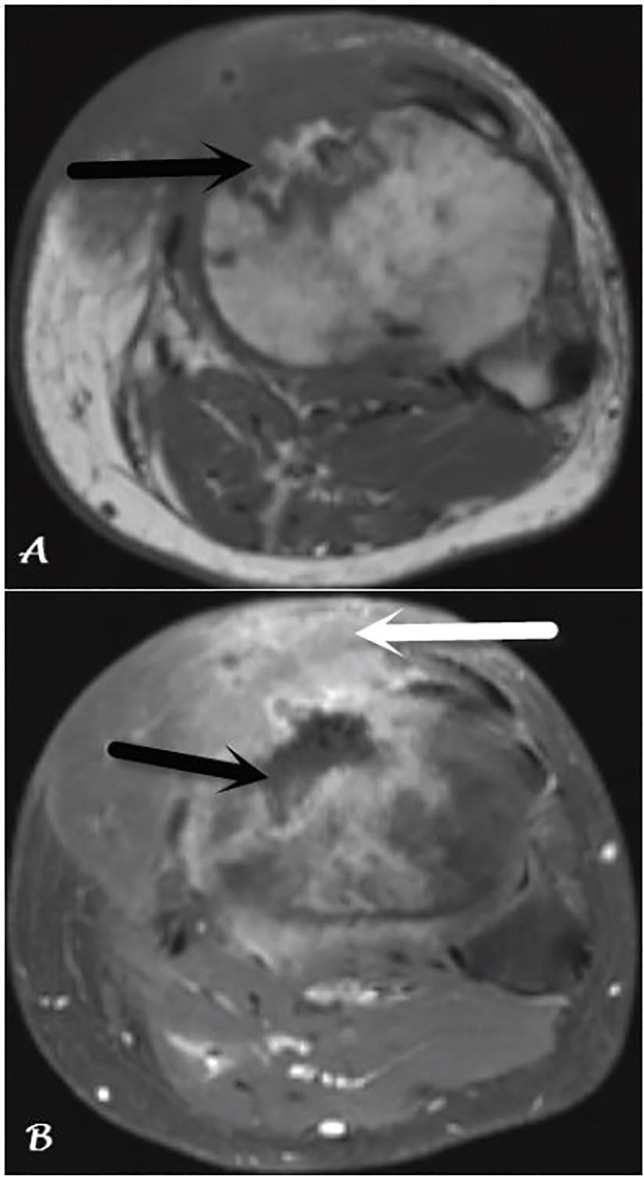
Magnetic resonance imaging of the proximal tibia: A) Axial T1-weighted image demonstrates abnormal heterogeneous marrow signal with associated anterior tibial cortical irregularity (arrow); B) Axial T1-weighted, contrast-enhanced image demonstrates a central region of non-enhancement (black arrow) representing devitalized bone. There is extensive surrounding enhancement of the bone and soft tissue (white arrow). These findings are consistent with osteomyelitis with surrounding soft tissue inflammation.
